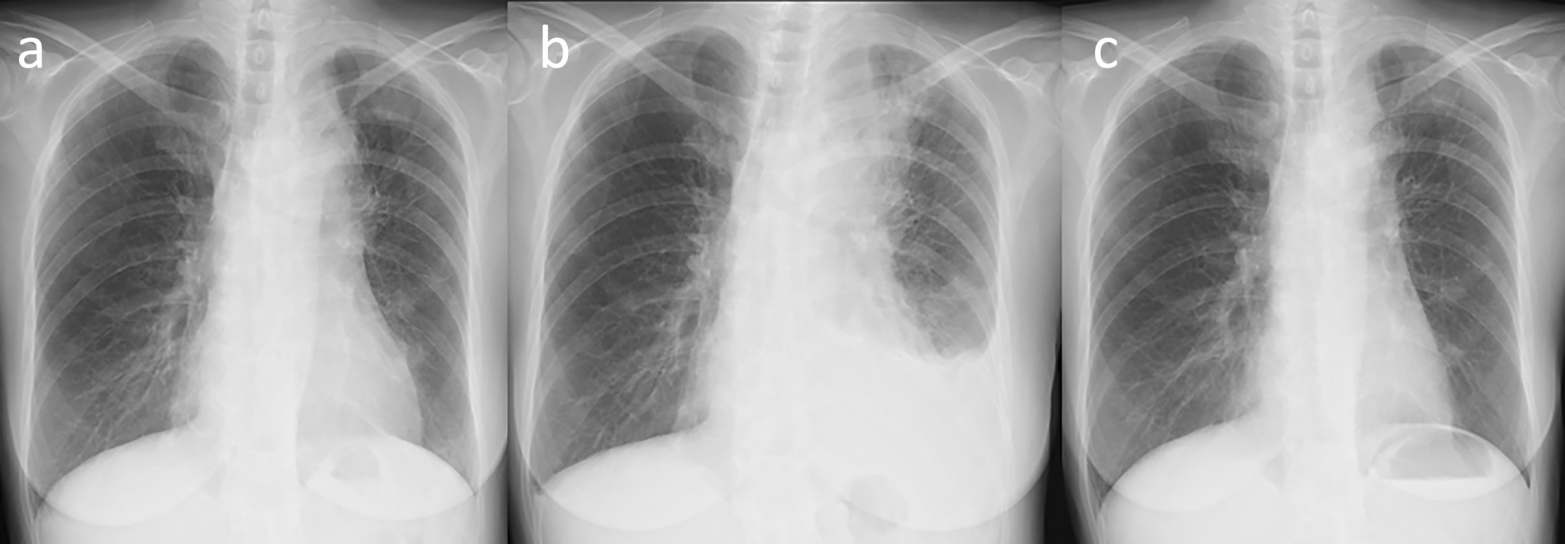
Serial chest radiographs before and after tarlatamab administration. (a) Baseline radiograph showing a left‐upper‐lobe mass without pleural effusion. (b) Day 7 after the first dose: Enlargement of the primary lesion and new left pleural effusion. (c) Day 12 after the first dose: The primary lesion is smaller than at baseline, and the pleural effusion has resolved.
